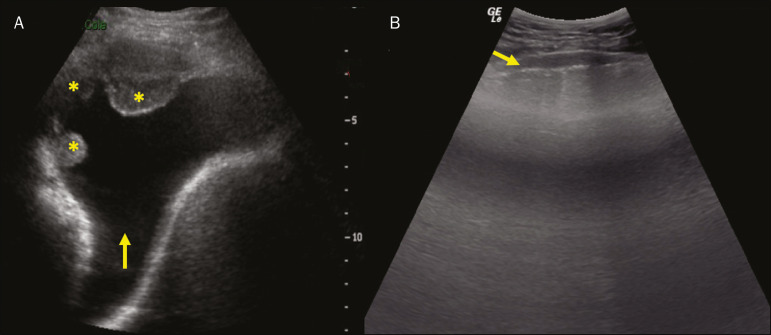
Other faces of the pleural effusion easily accessible on ultrasound. A: Multiple neoplastic nodules adhered to the pleura (asterisks) associated with pleural effusion (arrow). B: Pleural collection representing loculated pleural empyema (arrow).
